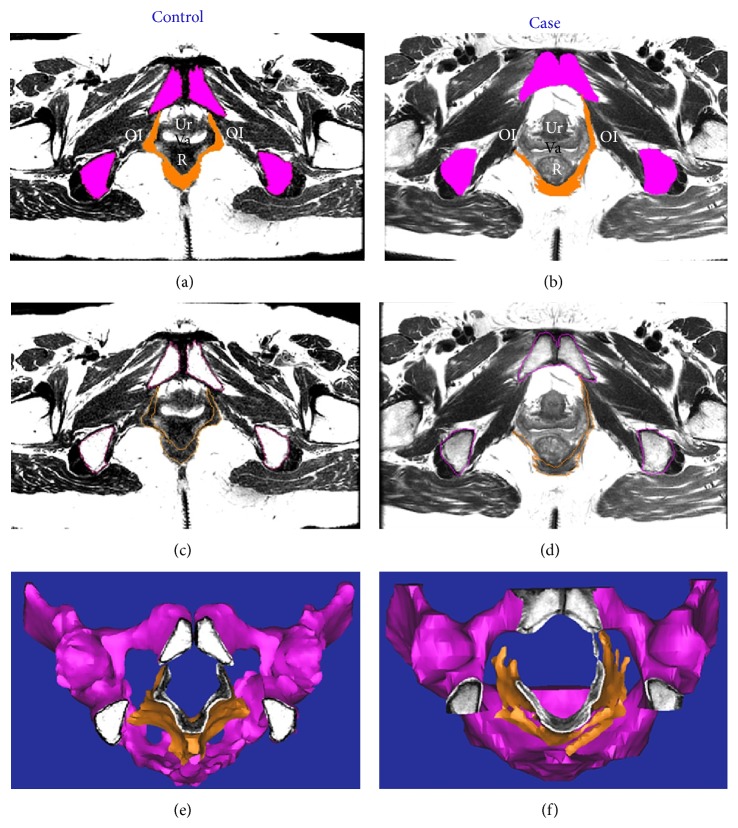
Axis magnetic resonance imaging (MRI) of a subject without ((a), (c)) and a patient with ((b), (d)) pelvic organ prolapse (POP). Top panel shows regions of the pelvis (magenta) and the levator ani muscle (orange). Middle panel shows outlines of the structures. Bottom panel shows a three-dimensional (3D) model on MRI. Note that the levator ani muscle of the control subject attaches to the pubic bones on both sides, whereas that of the POP patient has defects on the right side. Ur: urethra; Va: vagina; R: rectum; OI: obturator internus.
